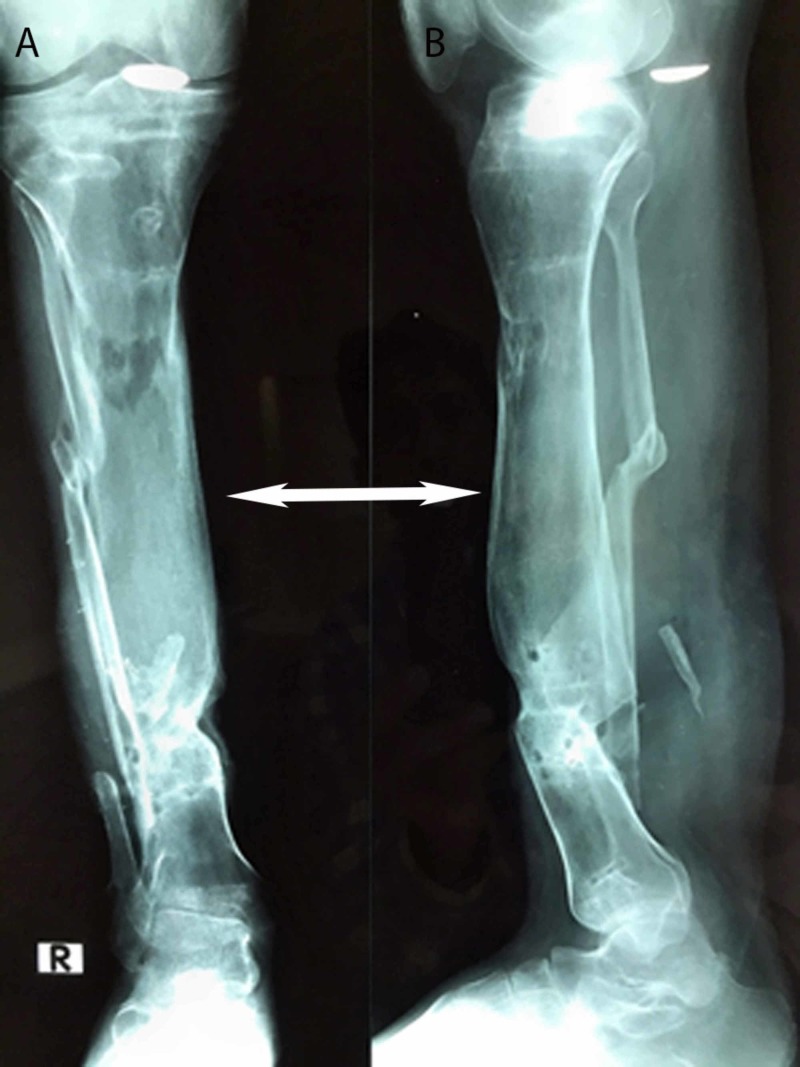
Postoperative plain radiographs of the same patient after removal of the Ilizarov frame, showing good regeneration of bone with an anteroposterior view (4A) and a lateral view (4B)
